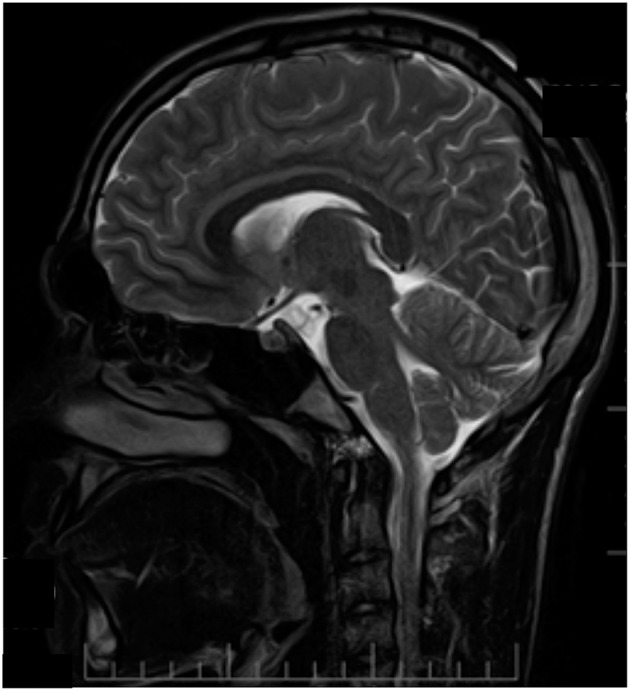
MRI examination (T2 weighted sagittal image) of patient S. No signs of atrophy in cerebellum. Patient information and technical text were closed.
